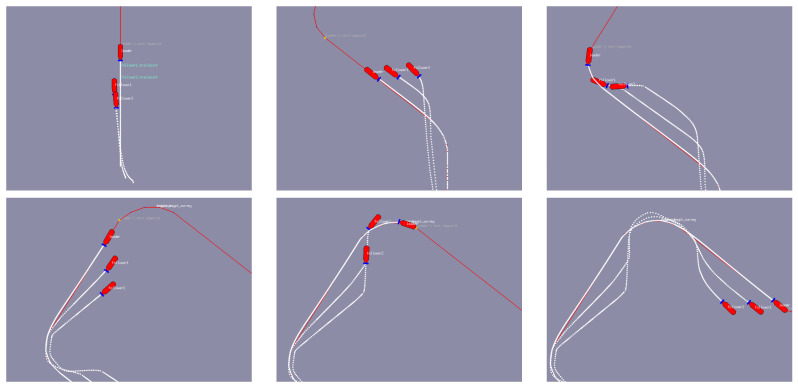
Example of NSCSUV-controlled vehicle behaviour on trajectory no. 1.
